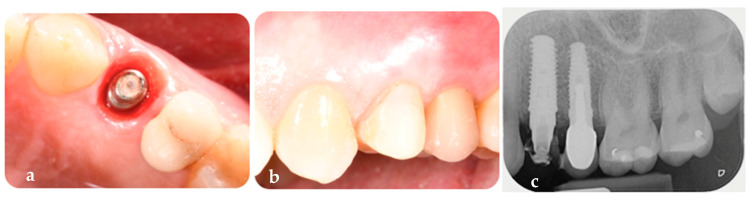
(a) Peri-implant osseomucosal seal achieved at 6-month follow-up with the definitive and customized FR abutment, FR coping, and provisional restoration. (b) Follow-up at 6 months in vestibular view with the provisional restoration in place. (c) Periapical radiograph at 6 months after implant placement, showing the FR abutment, FR coping, and provisional restoration.
